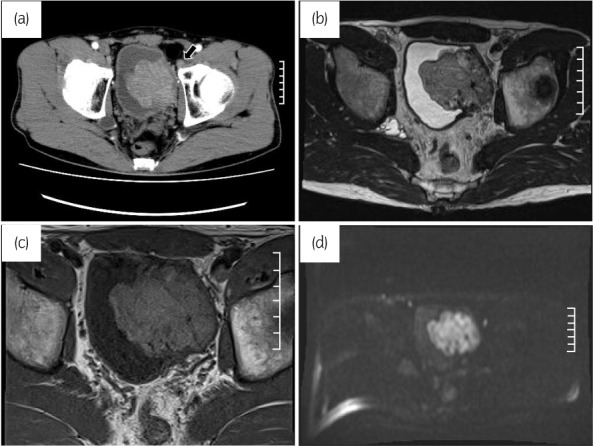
(a) CT revealed a 61 × 52 mm bladder tumor at the left side wall of the bladder and mild enlargement of the left external iliac lymph node (arrow). (b–d) MRI revealed a faint high signal on T2‐WI (b) and a high signal on T1‐WI (c) and DWI (d), which suggested extramural invasion.
